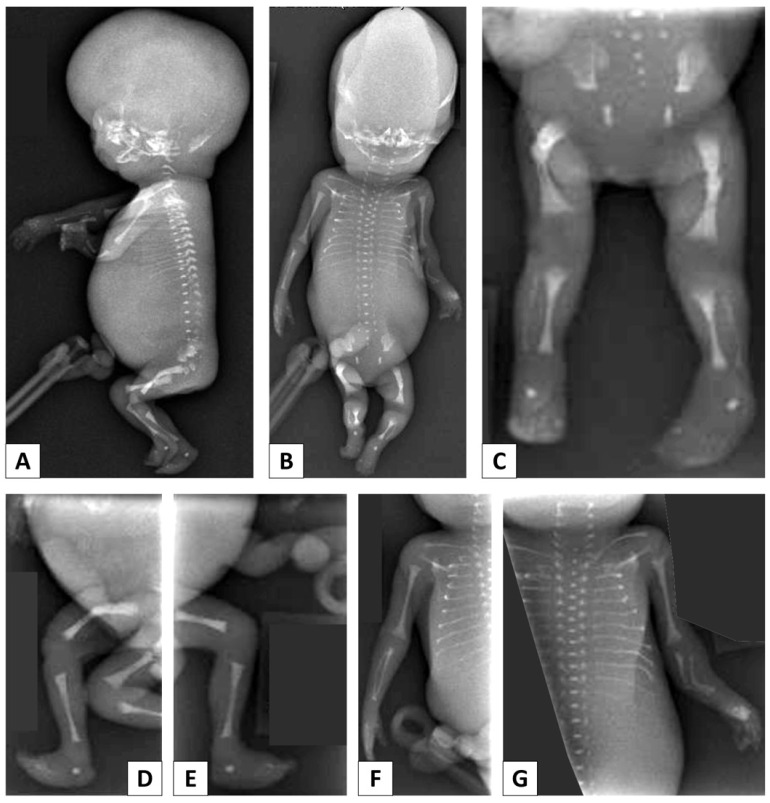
Clinical case #2: whole-body X-rays. (A–E) Reduced long bone length, thin long bones, bilateral femoral fractures with bowing. (F,G) Fractures with deformity of the left radius and ulna. (A,B,F,G) Gracile and irregular ribs, with fractures. (C,F,G) Bowing of the fibula and ulna. (B–G) Enlarged metaphyses of the tibia, femora and humeri.
